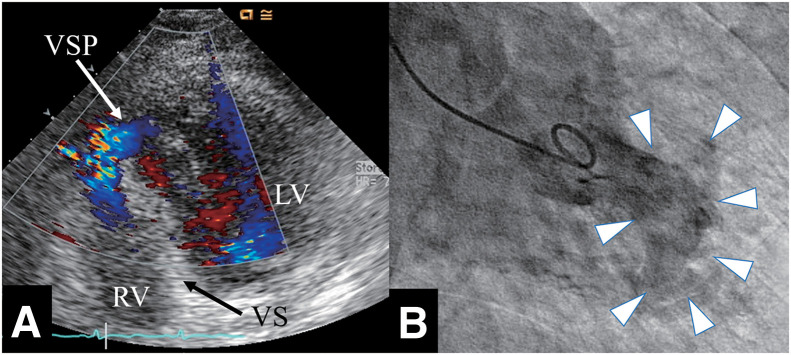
Transthoracic echocardiography and left ventriculography findings. Echocardiography reveals ventricular septal perforation at the apical ventricular septum (A). Left ventriculography shows ballooning of the left ventricular apical wall (arrowheads, B). LV, left ventricle; RV, right ventricle; VS, ventricular septum; VSP, ventricular septal perforation
